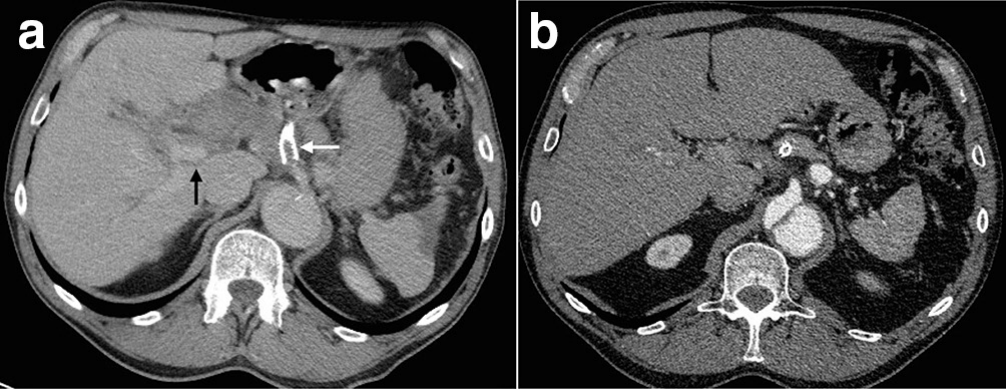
(a) Portal venous phase CT scan performed 1 month post-operatively showing the segment IV hepatic parenchymal changes have improved and the excluded aneurysm has started to reduce in size, with improvement of the portal vein compression (black arrow). The stent–graft remains occluded (white arrow). (b) 2 year follow-up arterial phase CT scan shows involution of the occluded aneurysm.
